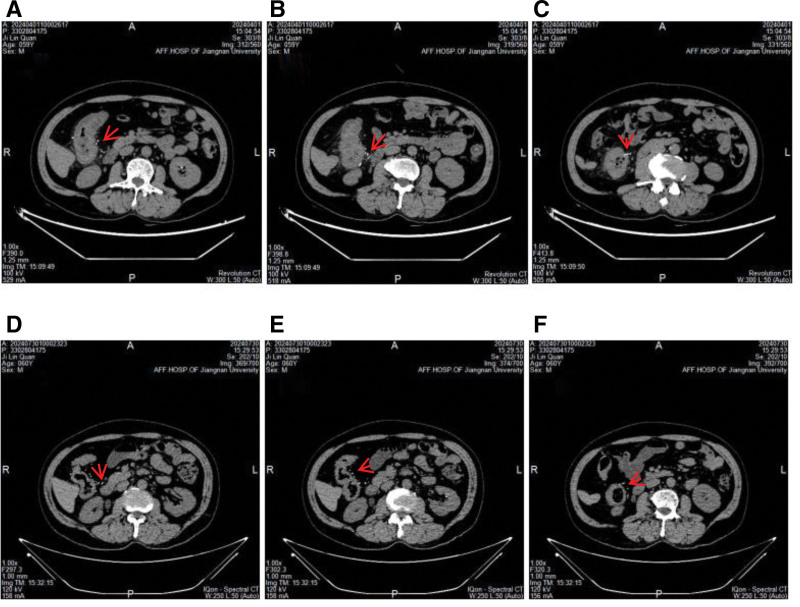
The CT imaging before and after treatment. (A–C) The CT imaging before treatment: calcification of mesenteric vessels, edematous thickening of the bowel wall of the ascending and transverse colon, and a slightly narrowed lumen. (D–F) The CT imaging after treatment: mesenteric vascular calcification as before, significant improvement of bowel wall edema in the ascending and transverse colon, and recovery of luminal narrowing. CT = computed tomography.
